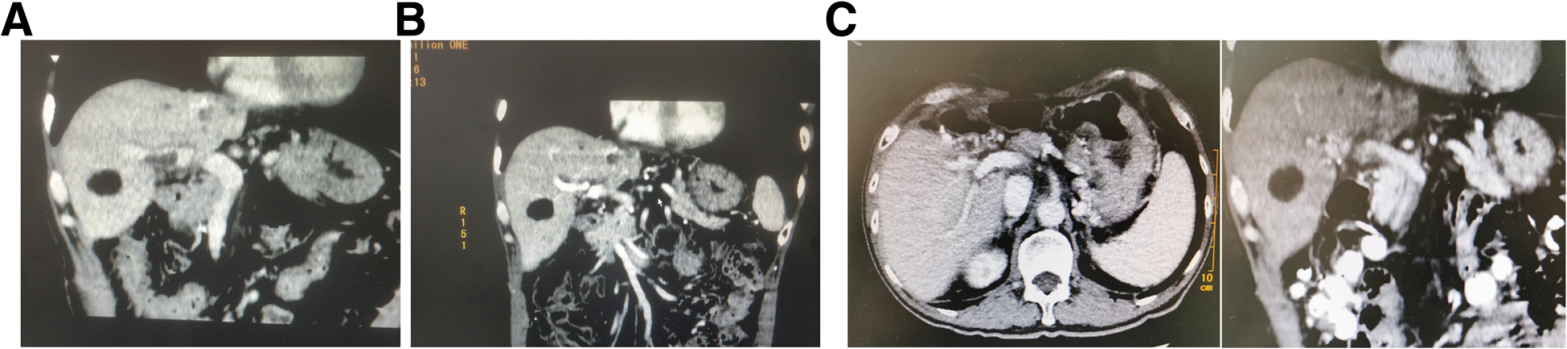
Representative computed tomography scan images after operation. a: A computed tomography scan images 2 months after the operation. Portal vein tumor thrombus reduced significantly. b: Computed tomography scan images 6 months after the operation. There were no portal vein filling defects, portal vein tumor thrombus was not overtly present in CT images. c: Computed tomography scan images 20 months after the operation. There was no tumor recurrence, portal vein tumor thrombus was not observable in the CT images
